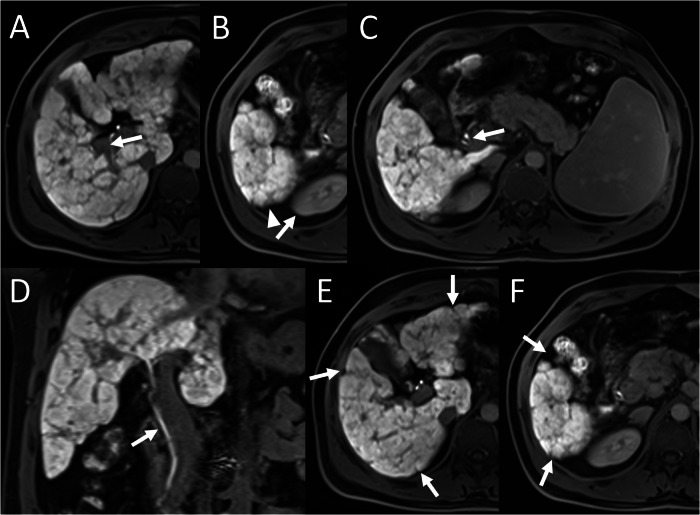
A 37-year-old male patient with chronic hepatitis B underwent abdominal MRI using gadoxetic acid. FLIS was calculated as 6/6 based on: (A) portal vein signal intensity (arrow) (score 2), (B) liver parenchymal enhancement (arrowhead and arrow) (score 2), and (C, D) contrast excretion into the extrahepatic bile ducts (arrow) (score 2). Despite the maximum FLIS, FibroScan^®^ indicated advanced fibrosis (F4). E, F The capsular retraction and heterogeneous parenchyma (arrows) reduced the FLIS-H to 6/8, better reflecting fibrosis severity
